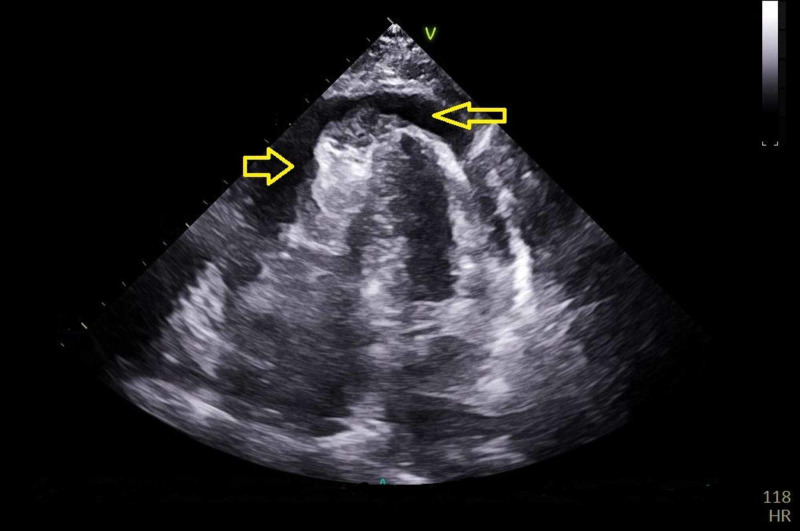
Transthoracic echocardiogram showing pericardial effusion
